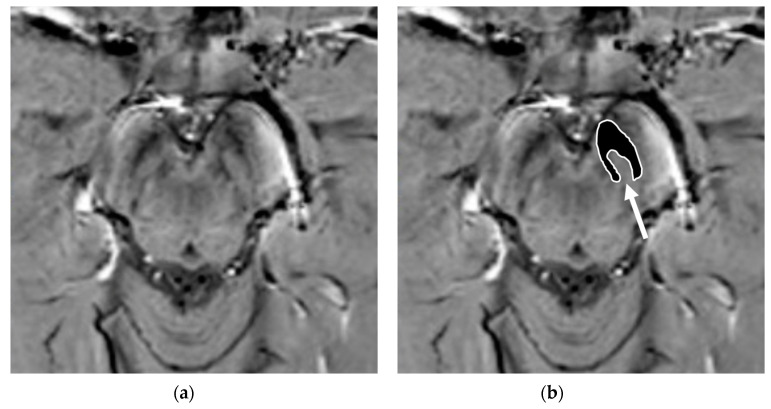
Susceptibility-weighted imaging (SWI) scan performed with a 3T MR scanner in a normally aging brain of a 65-year-old male who underwent a brain MRI examination for persistent headaches. A raw slice passing through the mesencephalon (a) and the same slice with superimposed highlighted SNc (white surrounded black ROI), thereby demonstrating the normal appearance of the nigrosome-1 (hyperintense area pointed by the white arrow) or swallow-tail sign (b).
